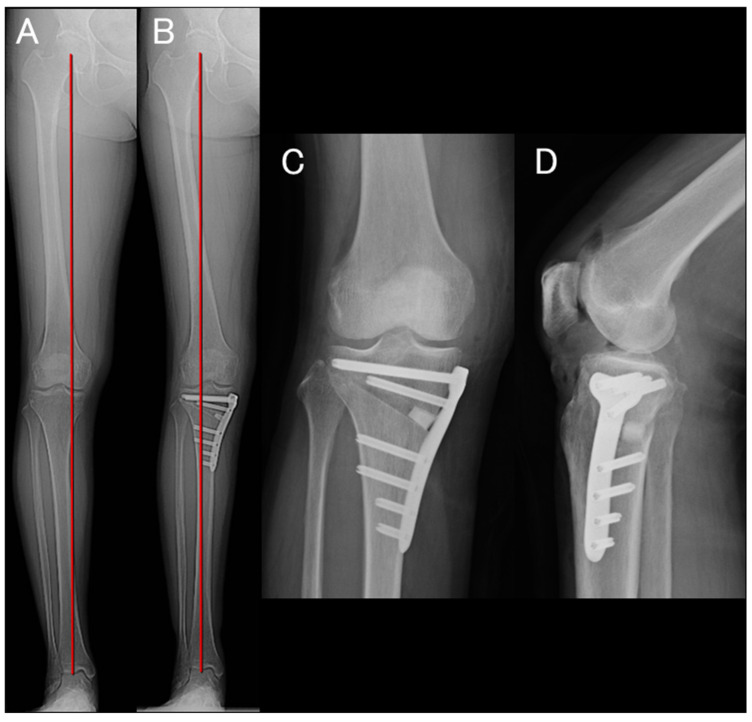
(A) Preoperative standing full-length anteroposterior radiograph of the lower extremity showing WBLR of 28.4%. (B) Postoperative standing full-length anteroposterior radiograph of the lower extremity showing WBLR of 63.4%. (C) Postoperative anteroposterior radiograph showing an 8 mm medial opening gap. (D) Postoperative lateral radiograph demonstrating that the bone substitute was inserted to provide firm posterior–medial support.
